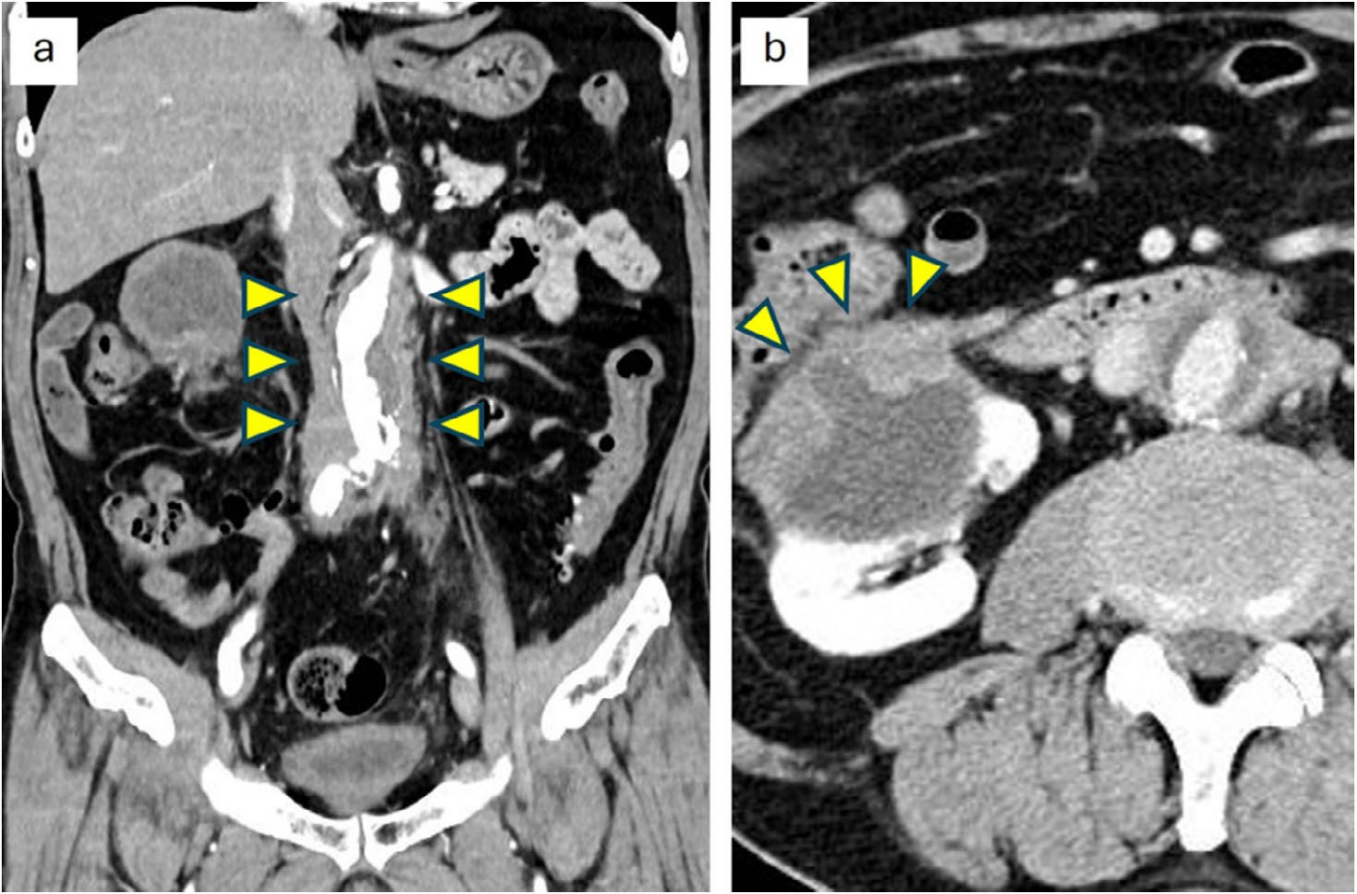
Contrast‐enhanced computed tomography imaging of a right renal cystic mass. Arrows represent the lesion. (a) Abdominal aorta atherosclerosis with periaortic fibrous thickening. (b) A 6.7‐cm cystic mass in the right kidney, characterized by thickened walls and mild enhancement, is classified as Bosniak category III.
